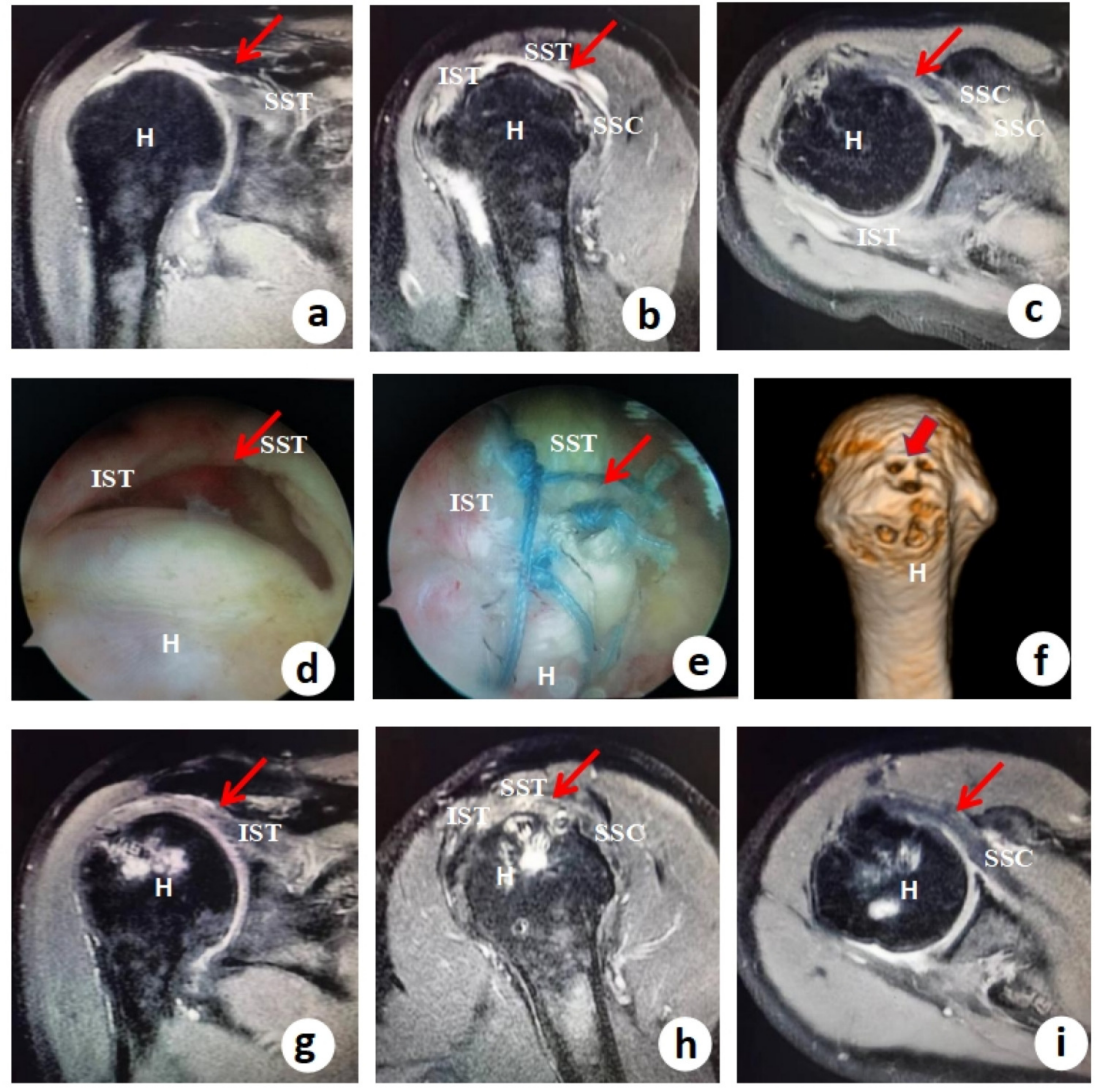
Preoperative imaging, arthroscopic findings, surgical repair, and postoperative outcomes in a massive rotator cuff tear treated with the TASS technique. (a–c) *Preoperative axial T2-weighted MRI scans* demonstrating a massive rotator cuff tear, with red arrows highlighting the involvement of the supraspinatus tendon (SST), infraspinatus tendon (IST), and subscapularis tendon (SSC). The high signal intensity (fluid/edema) within and around the tendons indicates significant tendon retraction and degeneration, consistent with a large, chronic tear. (d) Arthroscopic view of the massive “U-shaped” tear, a common pattern in extensive rotator cuff injuries where the tendon detaches medially from the greater tuberosity, leading to poor natural healing potential without surgical intervention. (e) Intraoperative arthroscopic view after completion of the stellate suture technique, showing secure knotting and suturing to reattach the torn tendons. This technique provides balanced tension distribution, reducing the risk of retear by minimizing focal stress points. (f) Placement of three suture anchors in the footprint area (greater tuberosity of the humerus) in a triangular anchor configuration, ensuring anatomical restoration of the rotator cuff insertion. The red arrows indicate anchor positions, which optimize biomechanical stability for tendon-to-bone healing. (g–i) *Postoperative axial T2-weighted MRI scans* confirming satisfactory tendon healing, with red arrows demonstrating the repaired SST, IST, and SSC tendons in continuity with the footprint. The absence of high signal intensity (fluid gaps) suggests successful biological healing without significant residual defects.
